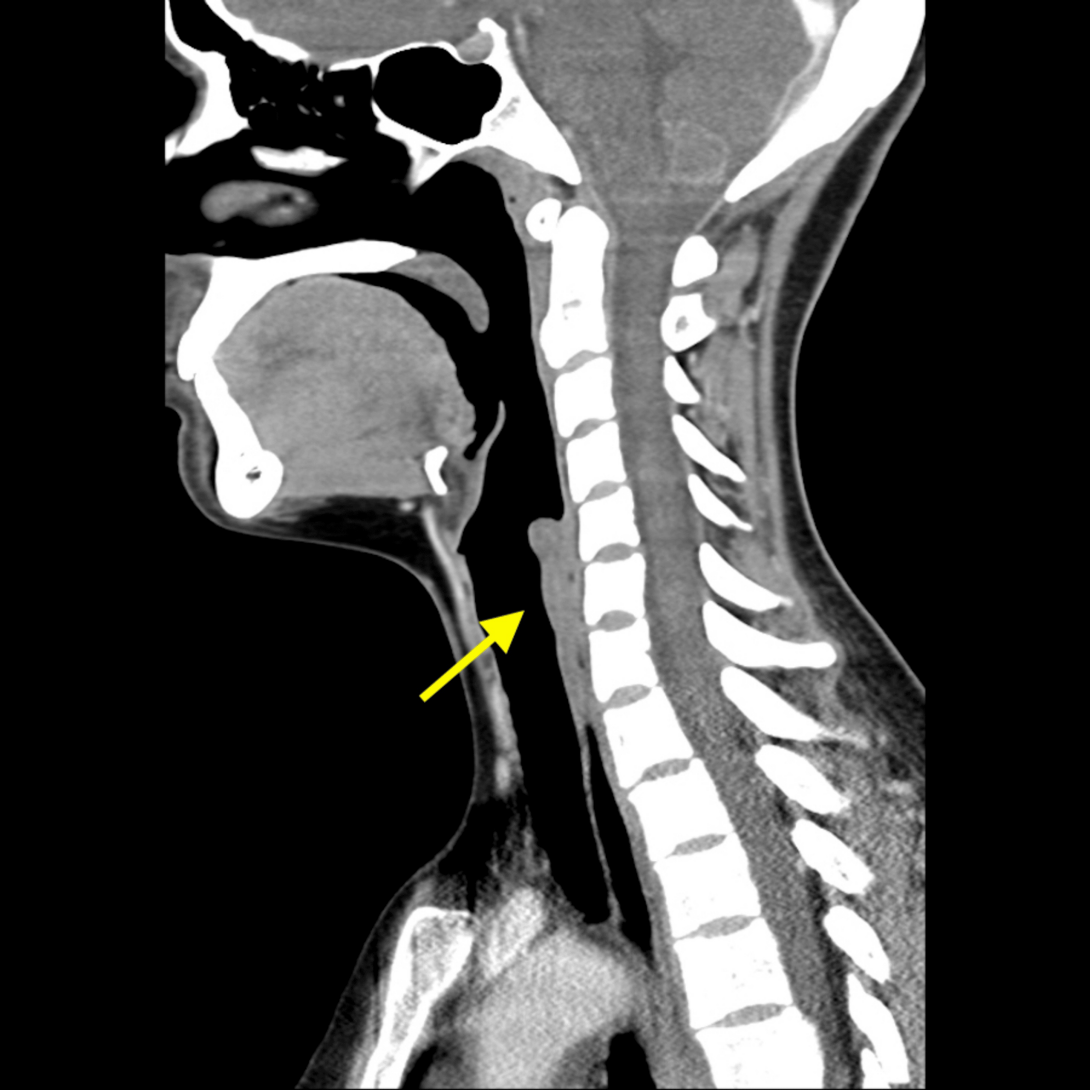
CT scan neck, sagittal section Arrow demonstrating a patent airway, normal epiglottis and symmetric prevertebral soft tissues. Cervical vertebrae are aligned normally with no evidence of mass, edema or lymphadenopathy.
